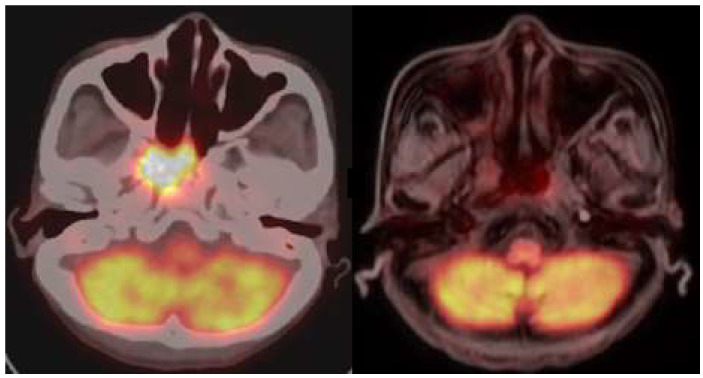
Imaging results for patient 5. (Left) The positron emission tomography (PET)/computed tomography (CT) image of patient 5 was taken before BNCT treatment. (Right) The PET/magnetic resonance imaging (MRI) scan displayed tumor recurrence in patient 5 following the complete treatment for three months.
